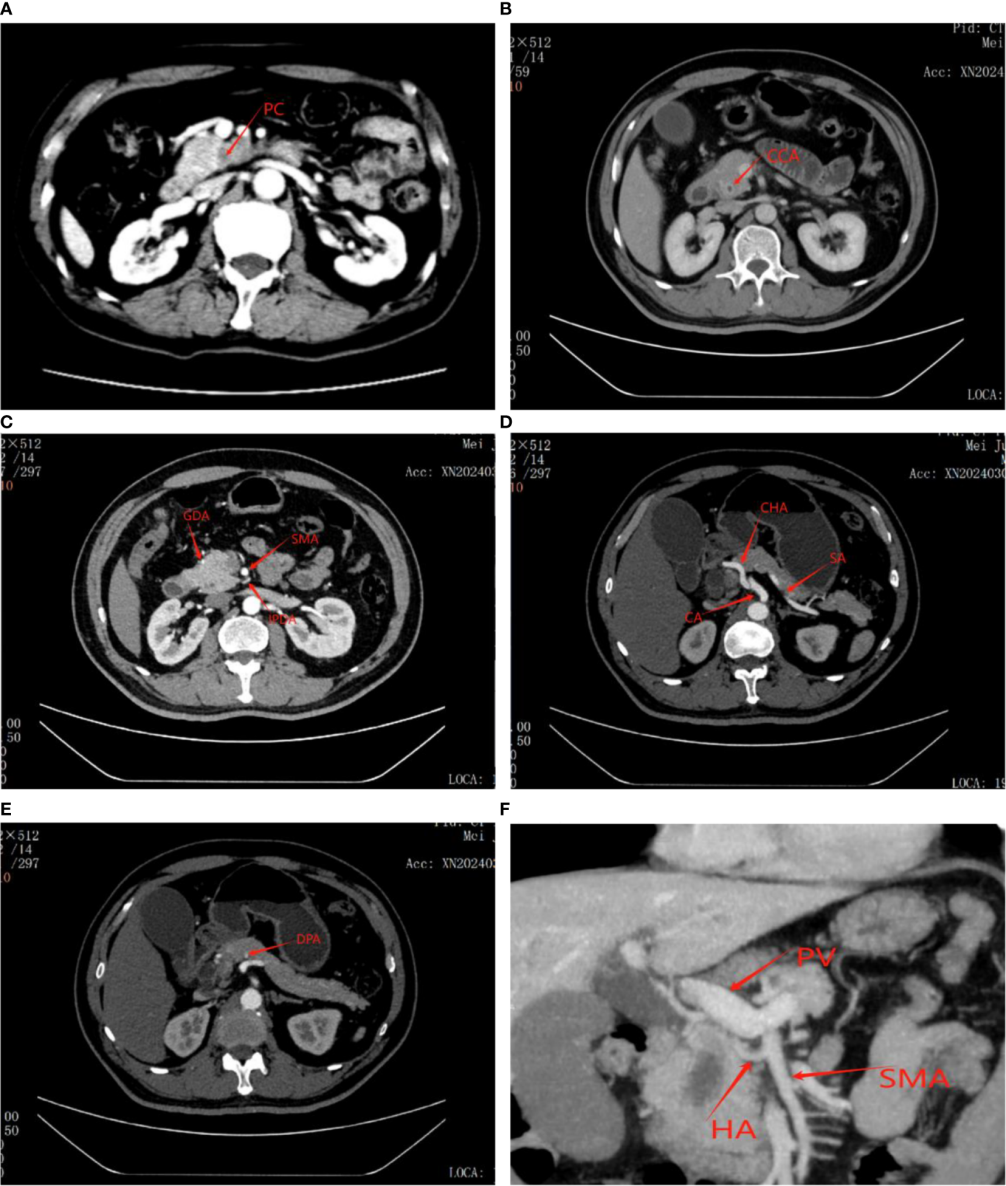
Figure showing tumor as well as its relationship to surrounding blood vessels and organs on CT scan before laparoscopic pancreaticoduodenectomy. (A) Pancreatic cancer. (B) Distal bile duct cancer. (C) GDA, Gastroduodenal artery; SMA, Superior mesenteric artery; IPDA, Inferior pancreaticoduodenal artery. (D) CA, Celiac artery; CHA, Common hepatic artery; SA, Splenic artery. (E) DPA, Distal pancreatic artery. (F) Accessory or replaced right hepatic artery (HA); PV, Portal Vein.
